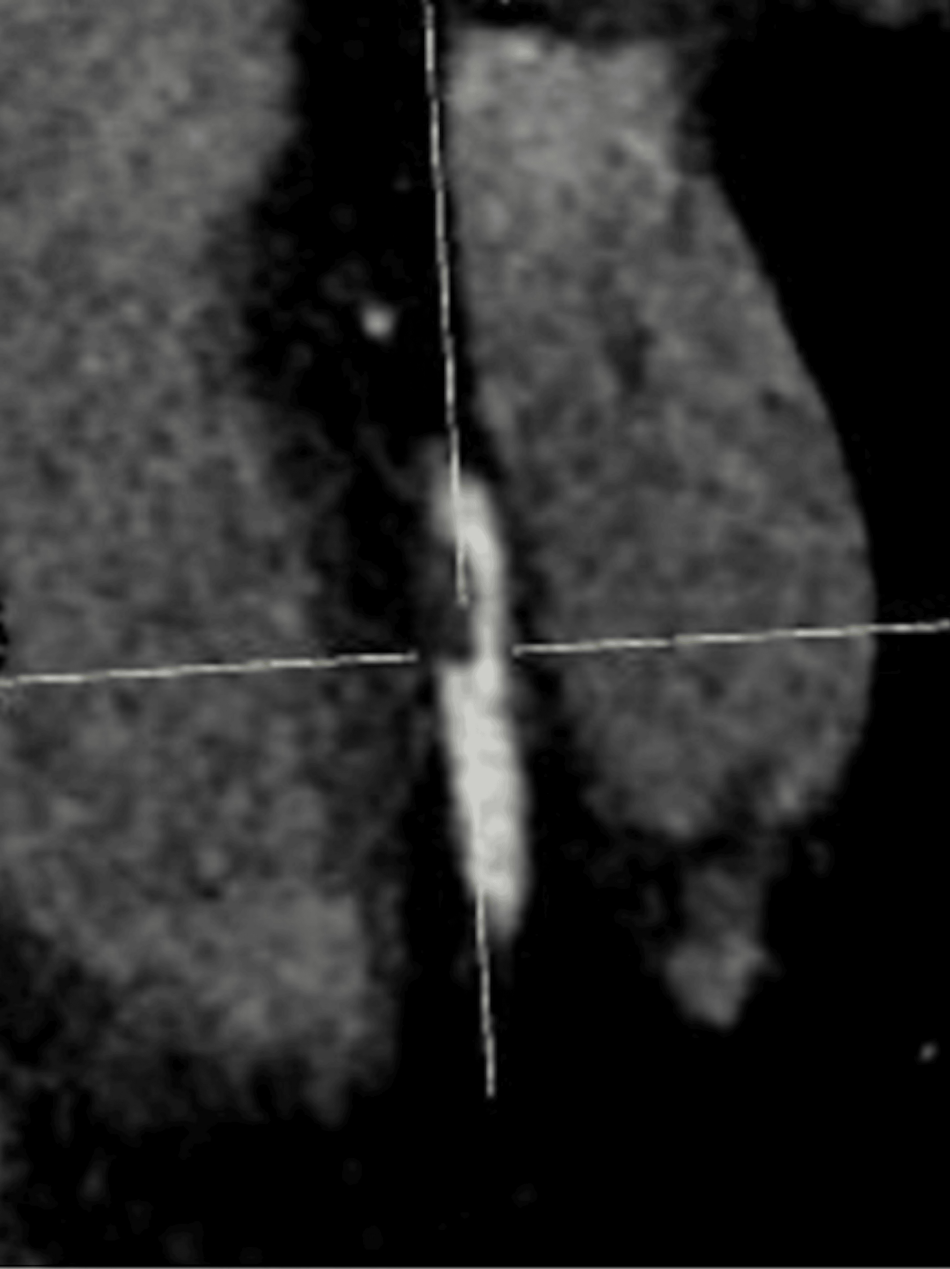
Double oblique view demonstrating the non-invasive nature of CCTA to visualize the proximal RCA dissection and hematoma RCA: right coronary artery; CCTA: coronary computed tomography angiography
